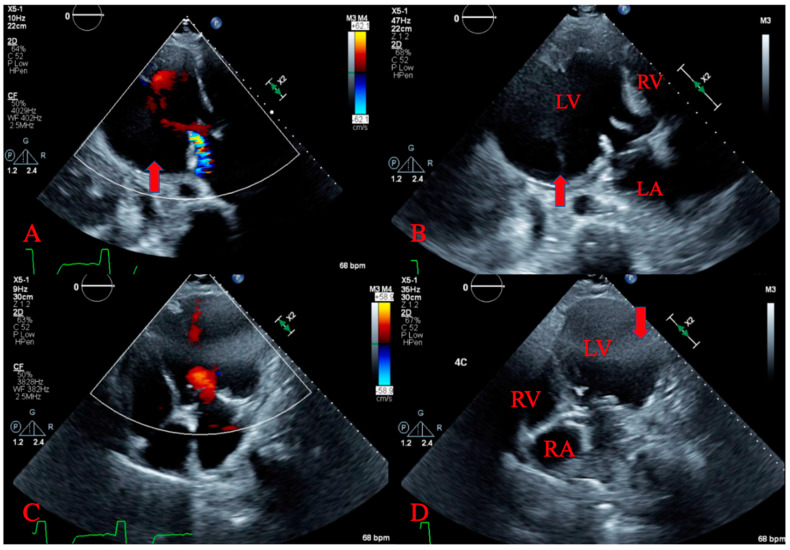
Transthoracic echocardiogram. (A,B) demonstrating left ventricular pseudoaneurysm with to and fro flow. (C,D) showing large loculated pericardial effusion.
